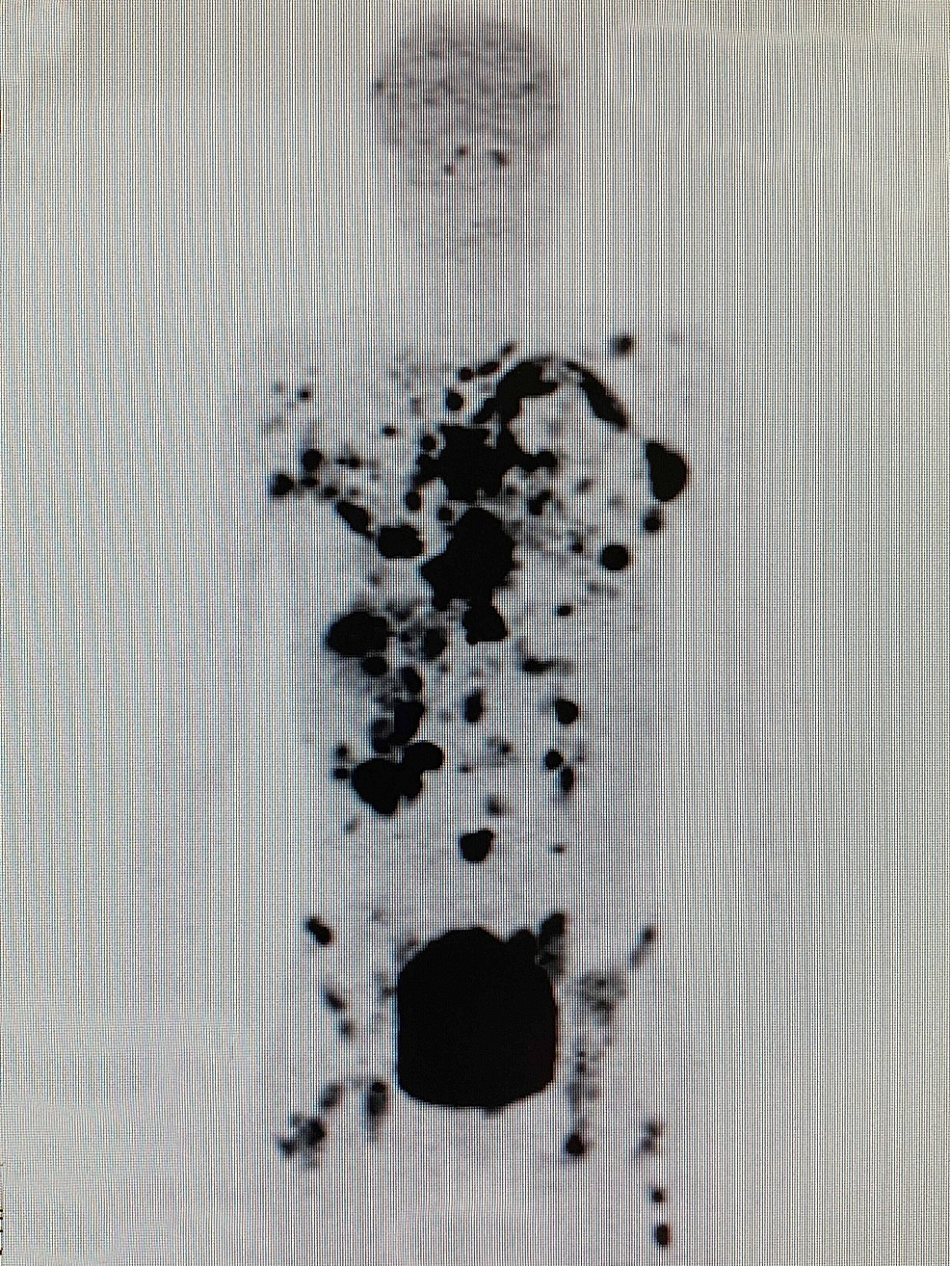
Whole body (18F)-FDG-PET/CT scan done before treatment on November 5, 2018 showing widespread multiple metastatic lesions at the lungs, mediastinum, liver, abdomen and bones. (18F)-FDG-PET/CT: 18F-fluorodeoxyglucose positron emission tomography/computed tomography
